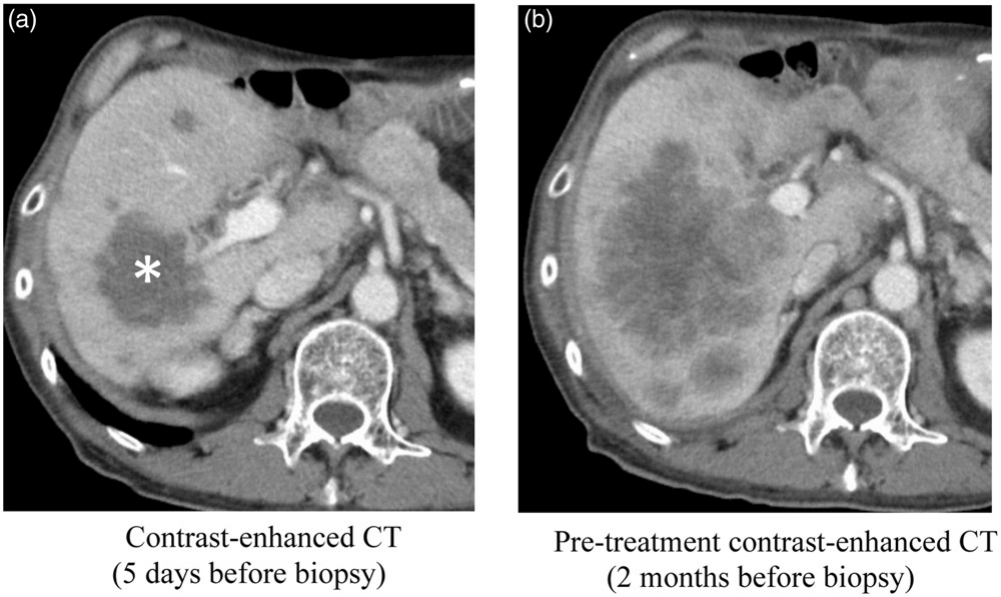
A case of failed NGS analysis. The patient is a man in his 60s with suspected liver metastases from bile duct cancer. (a) Contrast-enhanced CT 5 days before biopsy, after the anticancer medical therapy in a clinical trial. All the tumors demonstrated low attenuation representing hypovascular tumors. The largest mass in the posterior segment of the liver (asterisk) was selected for the target site of biopsy. Biopsy of five cores from various portions in the tumor was performed under ultrasound guidance. NGS analysis failed, and the pathological diagnosis was necrosis of the tumor. (b) Pre-treatment contrast-enhanced CT 2 months before biopsy. The diameters of the liver tumors are larger than that on post-treatment CT (a), and enhancement effects were seen in the periphery of the tumors.
